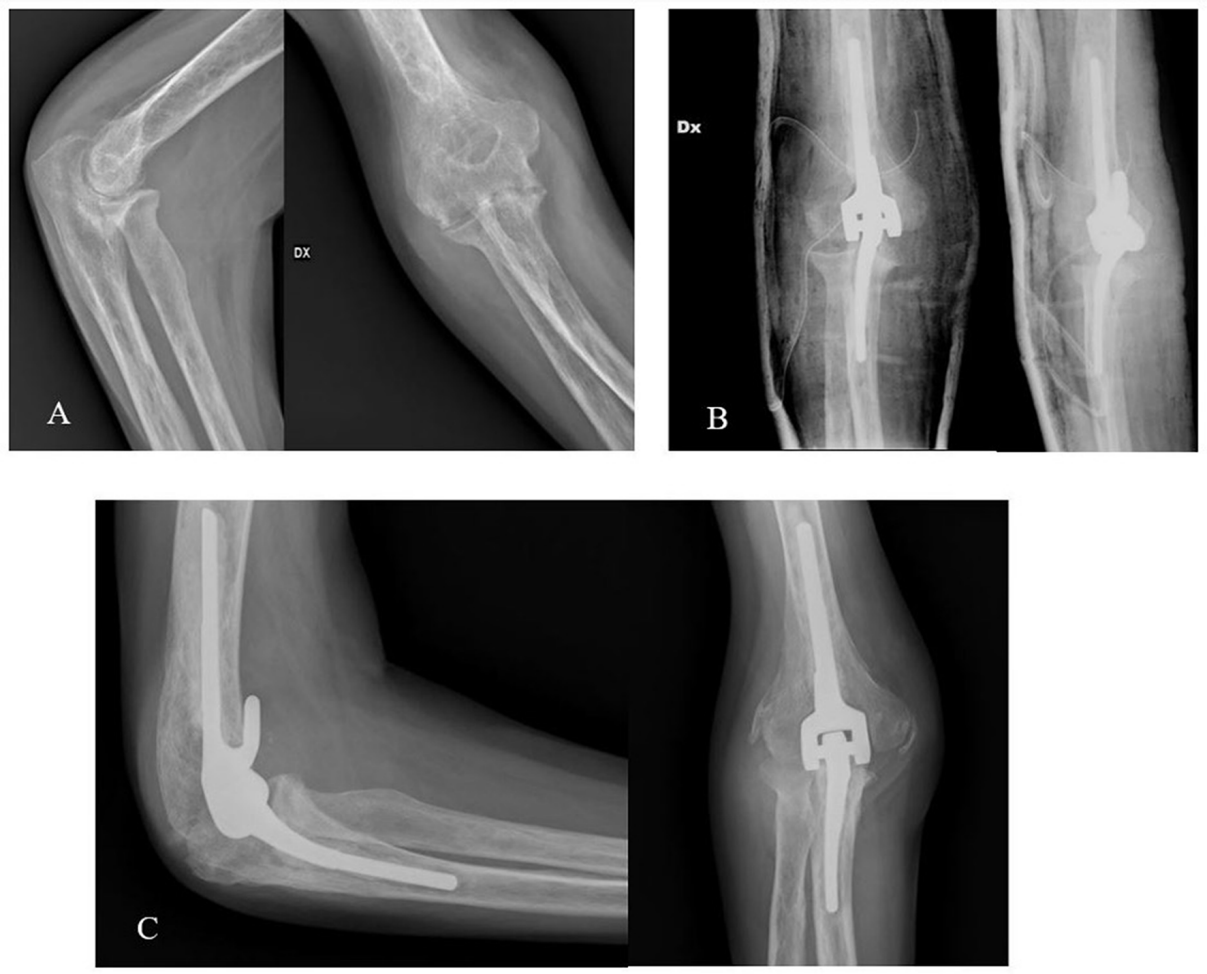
Sequence of radiographs from a clinical case of an elbow affected by primary osteoarthritis. (A) Preoperative x-rays; (B) Post-operative x-rays; (C) Follow-up x-rays one year after surgery
